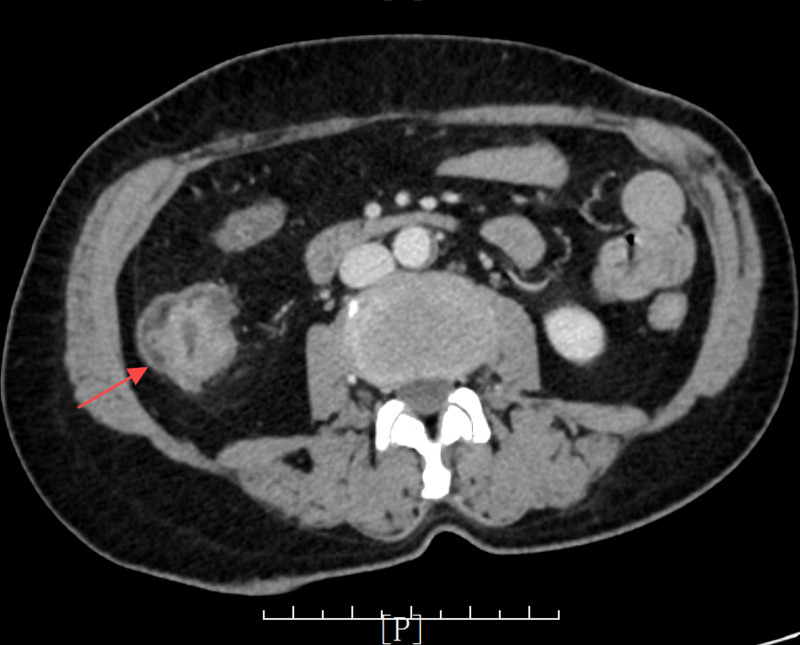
Computed tomography image (transverse section) shows ascending colon tumor (red arrow).
